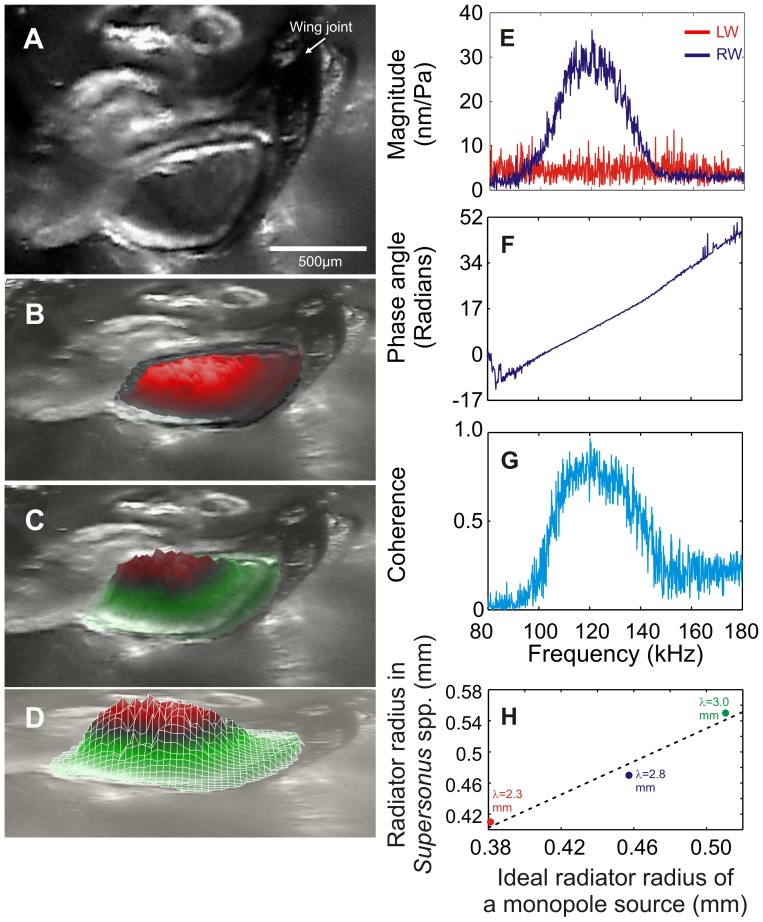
Wing resonance in *S. piercei* as measured with Laser Doppler Vibrometry. (A–D) Scanned area and defection shapes of the right wing (RW). A and B show the orientation image relating wing topology to the position of the scanning latice. (C, D) Area scans of mirror deflection at best response (122 kHz in this species). Wings scanned in normal position close to the body. Note how the mirror membrane strongly deflects while the rest of the wing veins and folded membranes rest in position. (E) Displacement and resonances of the left wing (LW) and RW. (F) Phase gain response of RW vibration. (G) Coherence across the frequency range measured for the RW response. (H) Expected and observed radiator size optimal for the frequencies used by *Supersonus* spp.
